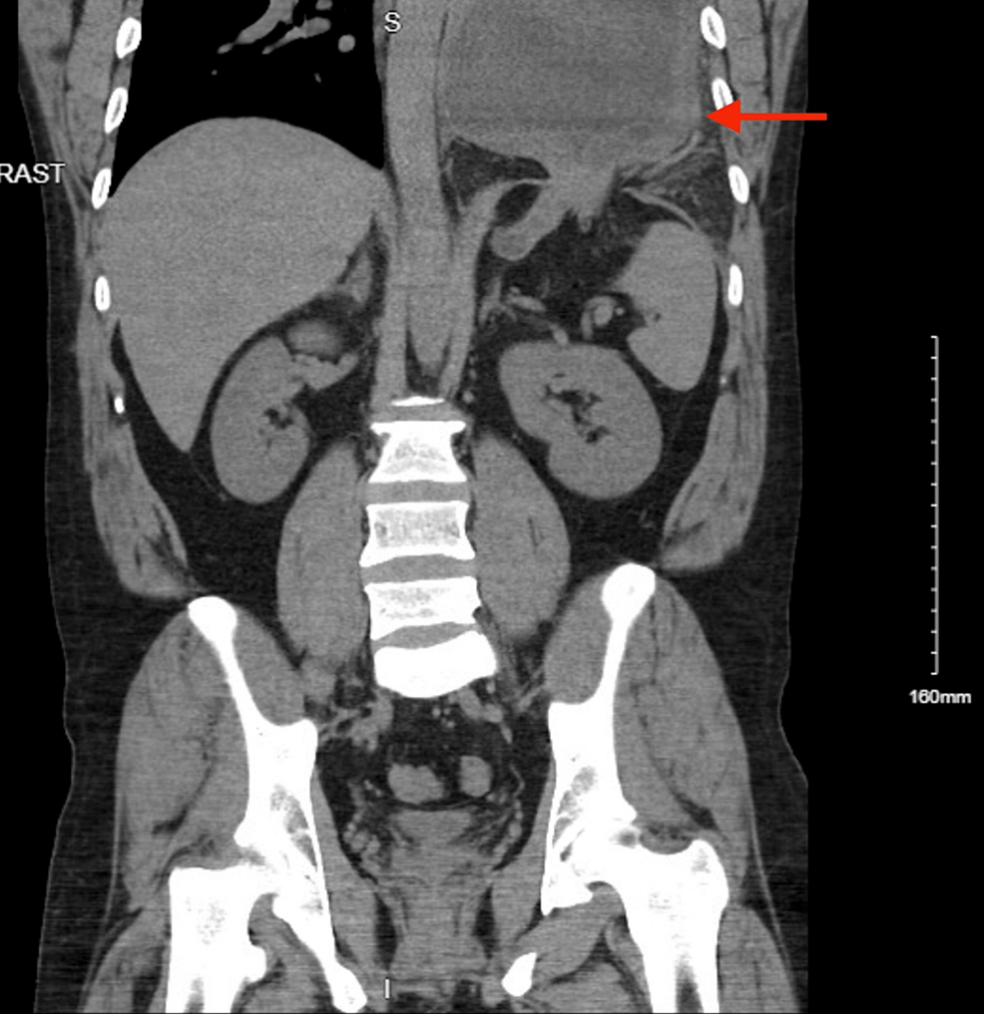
Coronal view on CT of the abdomen/pelvis points to the herniated stomach.
